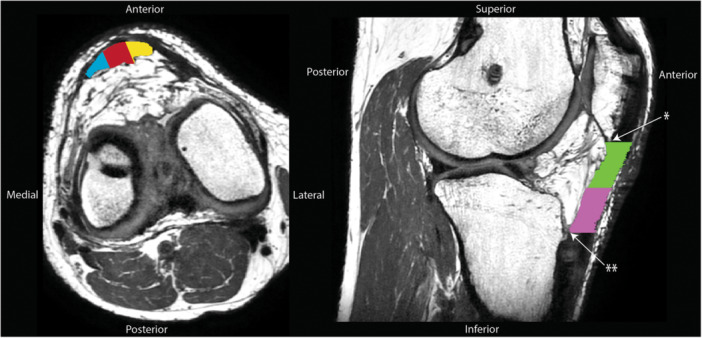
Proton density MRI overlaid with masks of the five patellar tendon boundaries for separating regions of interest. The free tendon was segmented from the distal end of the patella (*) to the attachment on the tibia (). The tendon mask was then separated into five regions: (1) medial, (2) central, and (3) lateral regions by trisecting the tendon longitudinally into equal widths (left) and (4) proximal and (5) distal regions of equal length (right).
